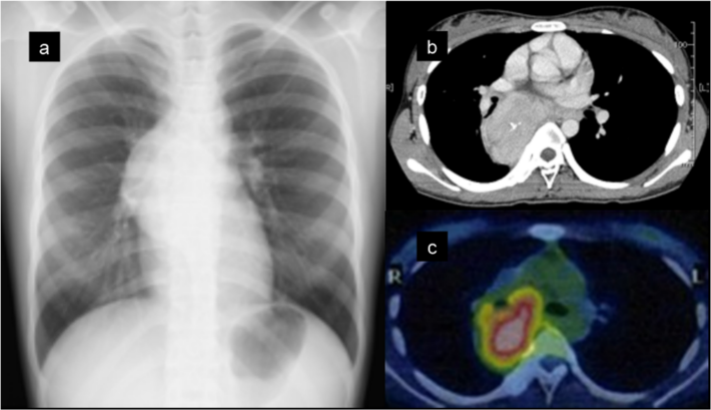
Preoperative imaging studies. Chest X-ray film showed a large para-cardiac mass in the right mediastinum (a). Contrast-enhanced computed tomography showed a heterogeneous attenuation within the mass and a focus of coarse calcification (b). 18-fluorodeoxyglucose (FDG) positron emission tomography/computed tomography showed moderate FDG uptake within the mass (c). *Pink-colored area* indicates that the maximum standardized uptake value was 4.0 or more
